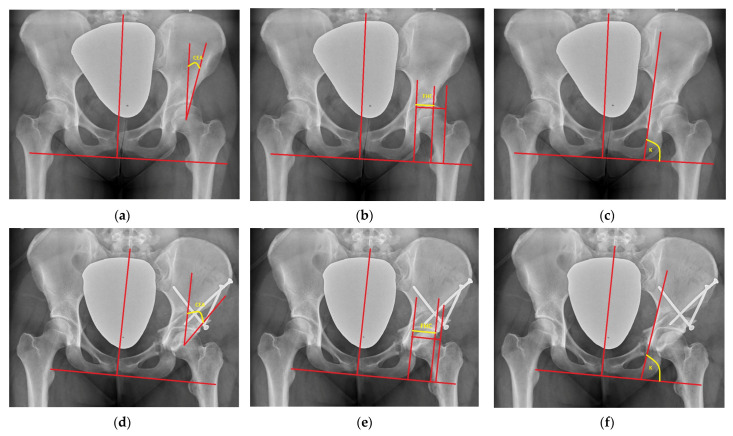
(a–c) Radiographic measurements of CEA (10°), FHC (52%), and K ilioischial angle (87°) preoperatively; (d–f) radiographic measurements of CEA (28°), FHC (80%), and K ilioischial angle (82°) postoperatively.
